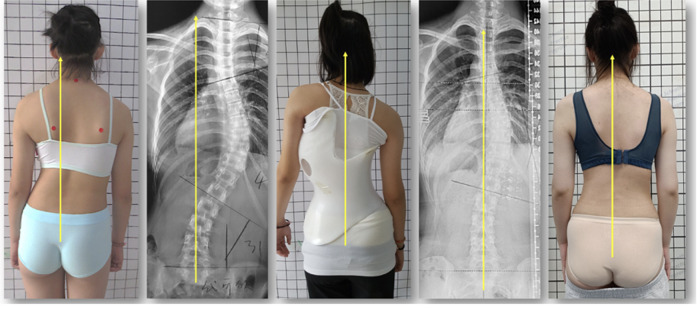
Patient with an AIS (pattern 3CH according to the ALS-classification, born in 2005. In August 2017 the thoracic curvature was 48 degrees. The x-ray taken one year after full weaning of the brace (2022) showed a thoracic curvature of 28 degrees with a marked realignment of the formerly vastly decompensated trunk.
